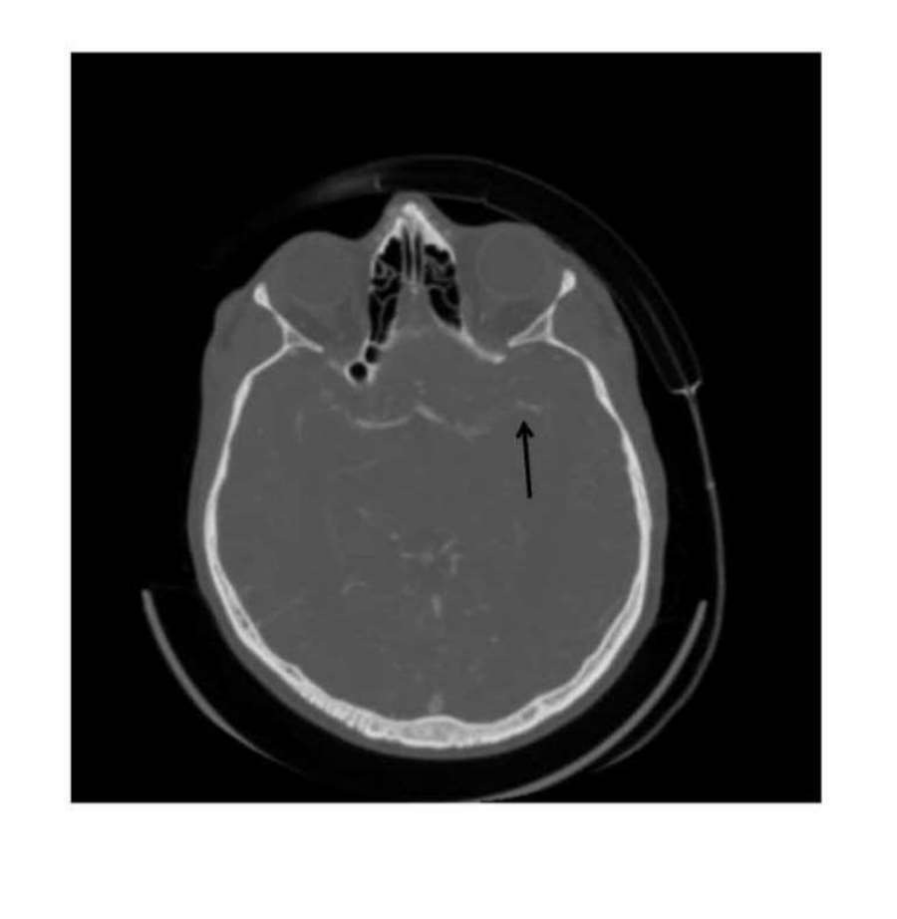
CT angiogram showing the cut-off of blood supply at the M2 part of the left middle cerebral artery Computed tomography angiogram (CT) showing the cut-off of blood supply at the M2 part of the left middle cerebral artery
